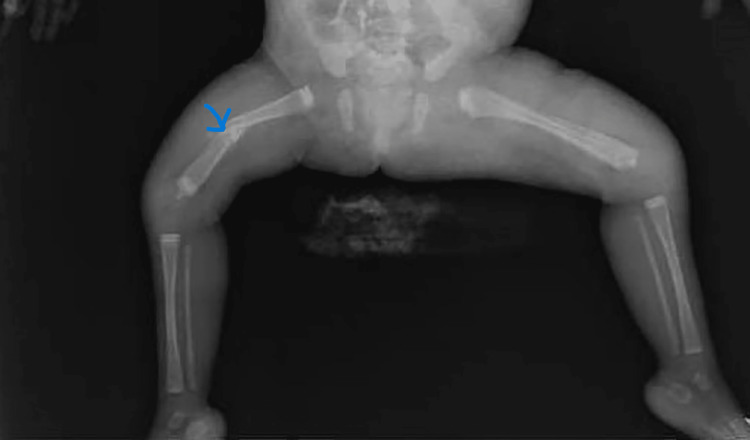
X-ray showing fracture of the right femur of case 1
